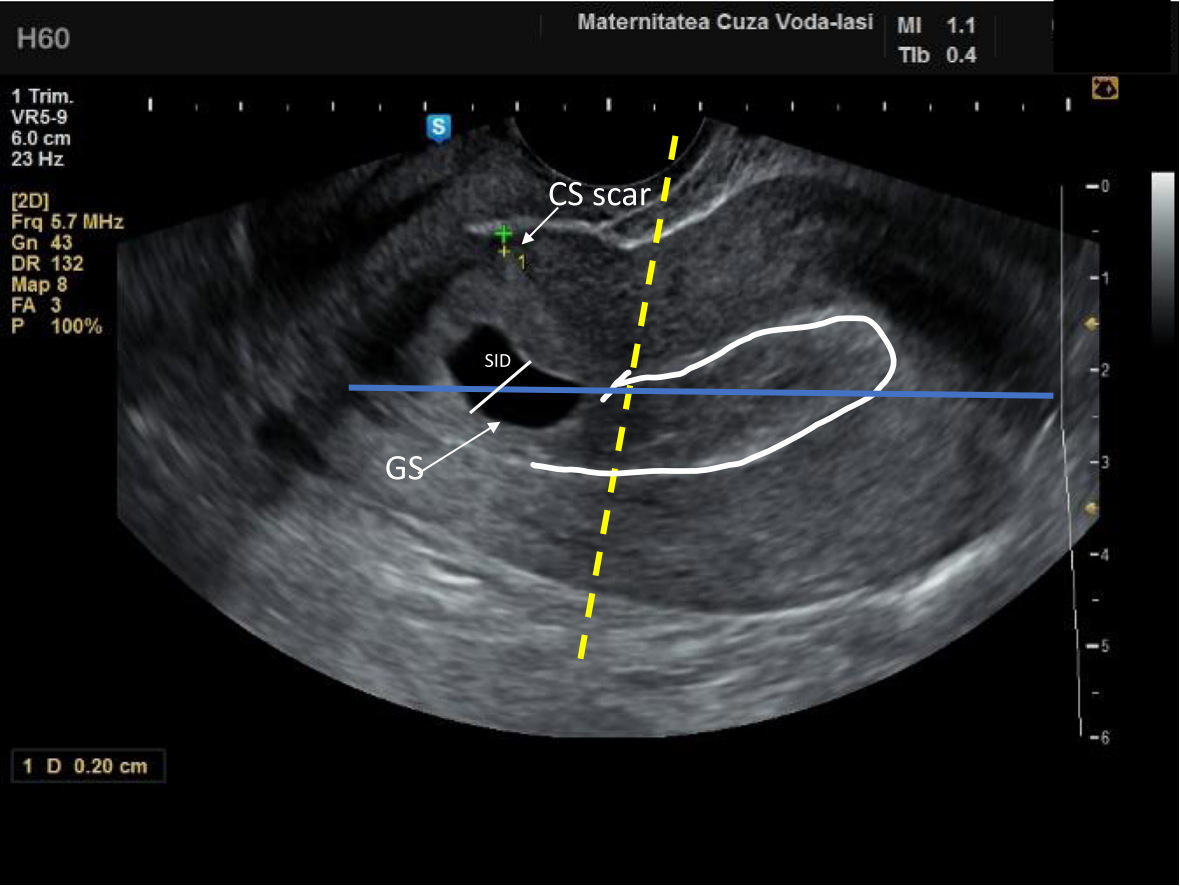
Sonographic differential diagnosis between intrauterine pregnancy and caesarean section scar pregnancy in the early first trimester. Interrupted yellow line on a sagittal section of the uterus – imaginary line dividing uterus in two portions described by Timor-Tritsch et al. [9]. Continuous blue line on a sagittal section of the uterus – imaginary endometrial line connecting internal cervical os with the uterine fundus described by Cali et al. [14]. The gestational sac is situated below the yellow line, implanted at the level of CS scar. Less than two thirds of the SID line are above the endometrial line, towards the anterior uterine wall. The uterine cavity is empty. The myometrial layer thickness is 2 mm. CS, caesarean section; GS, gestational sac; SID, superior-inferior diameter of gestational sac
